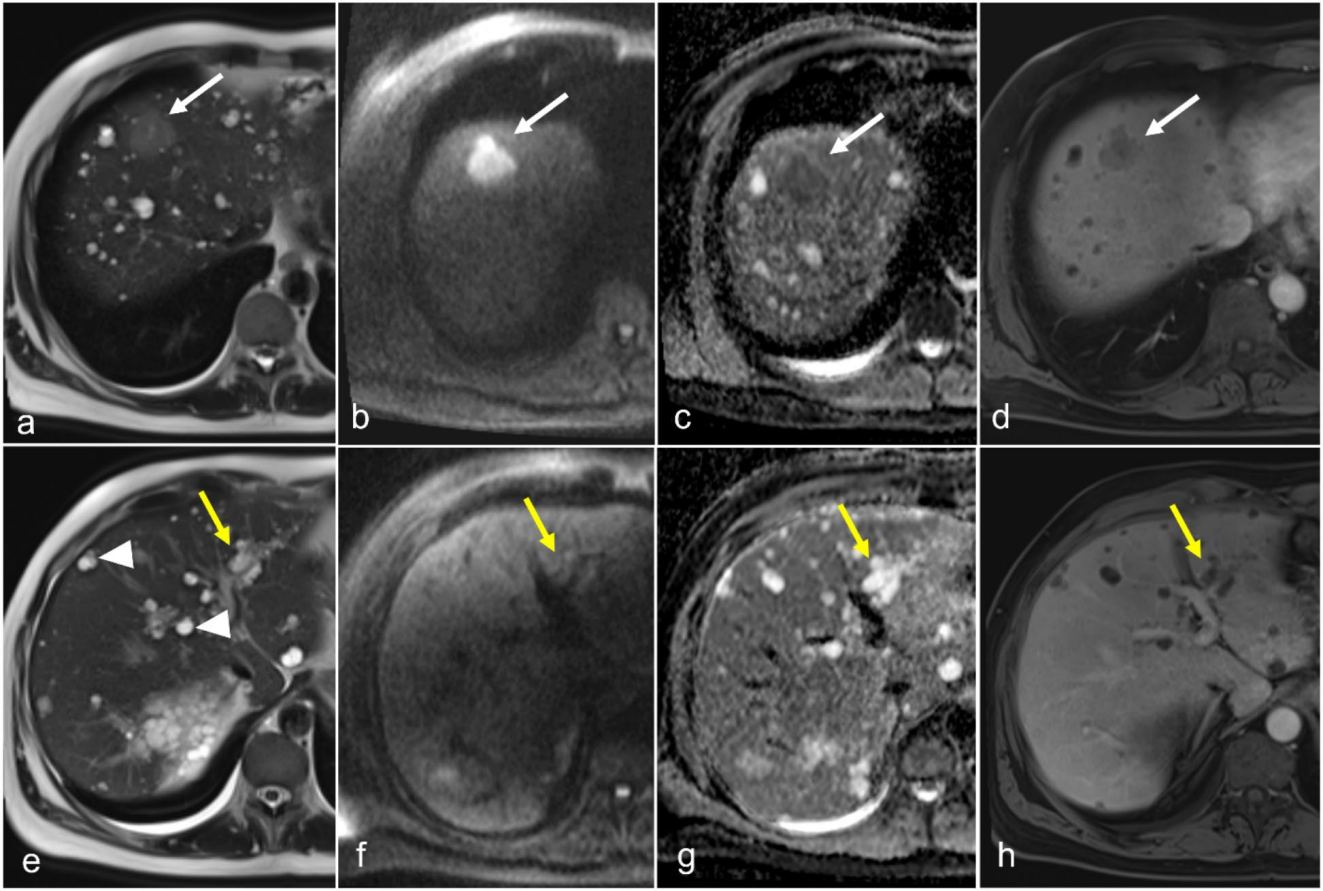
Cystic metastasis in a patient with a history of autosomal dominant polycystic kidney disease and sigmoid carcinoma with solitary liver metastases. Axial T2-weighted images (a) demonstrate a mild T2-weighted hyperintense mass (arrow) with focus of central necrosis in hepatic segment 8 near the dome. The mass demonstrates increased signal intensity on diffusion-weighted images (b) and appears dark on the ADC maps (c) representing restricted diffusion. On late arterial phase postcontrast T1-weighted imaging (d), the mass demonstrates mild central enhancement with thickened and irregular peripheral enhancement (arrow). The mass (white arrows) demonstrates a more distinctive feature from liver parenchymal cysts (arrowheads) and the peribiliary cysts in the left hepatic lobe (yellow arrows). The cysts demonstrate more intense T2-weighted hyperintensity (e); no restricted diffusion (f, g); and thin, smooth peripheral enhancement (h). The segment 8 mass is a biopsy-proven adenocarcinoma metastasis
